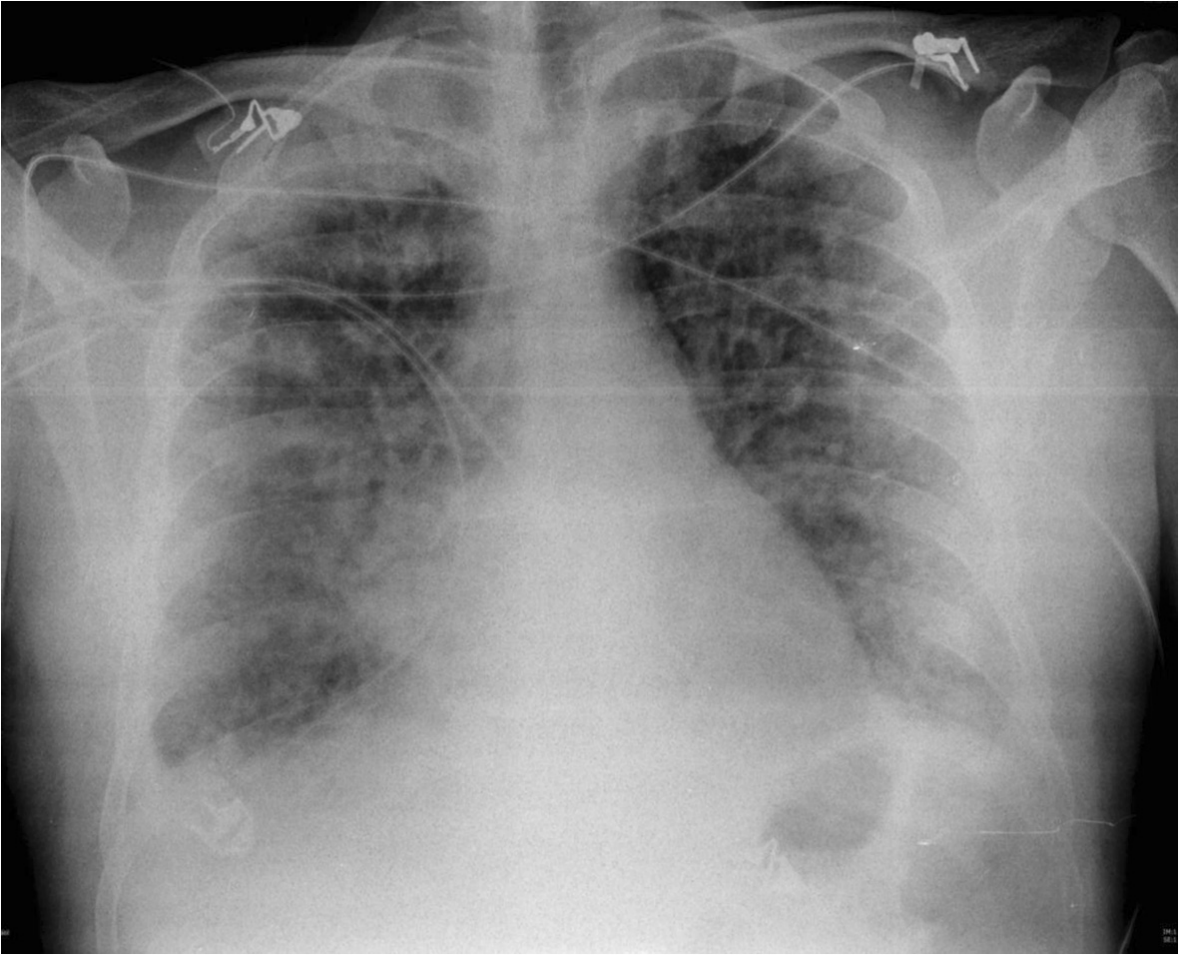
Anteroposterior chest radiograph after nailing. Note the diffuse bilateral infiltrates.
